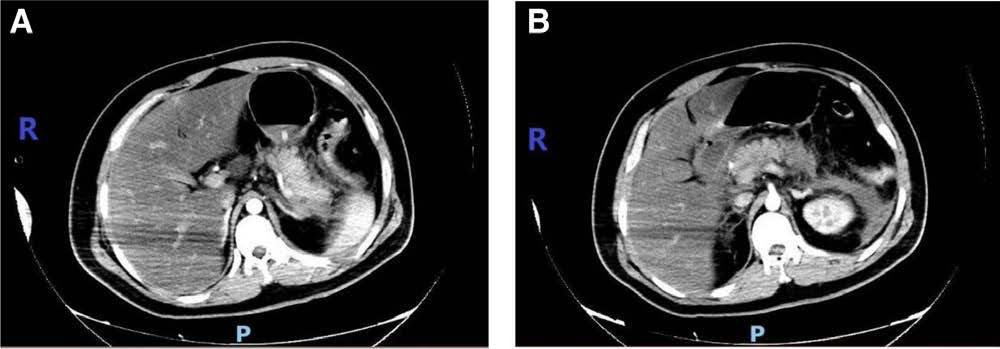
Enhanced CT of the upper abdomen: pancreatic edema, necrotic exudation around the pancreas and around the left kidney, fatty liver or hepatic edema. CT = computed tomography.
